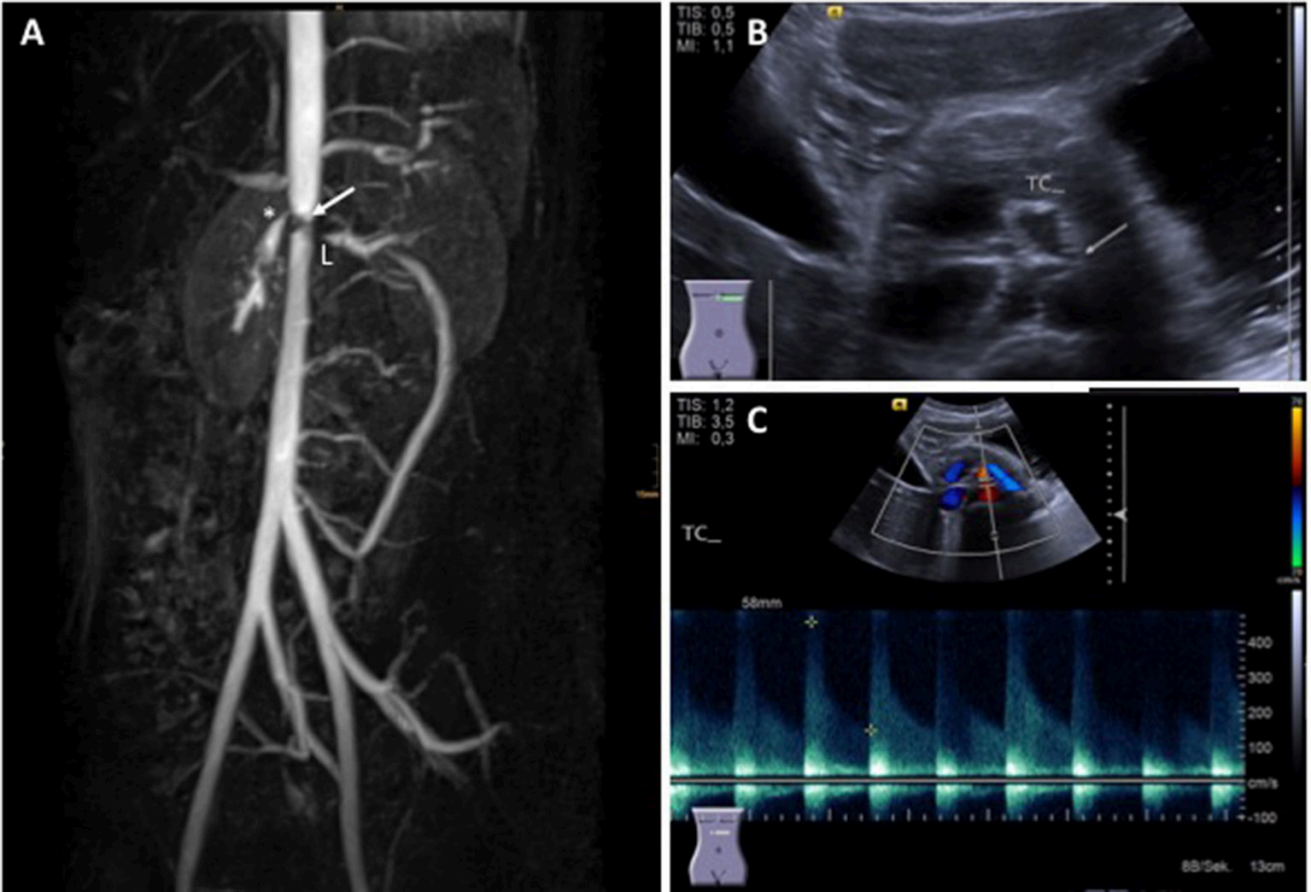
Takayasu arteritis in a 12-year-old girl. (A) MRI angiography showing significant stenosis of the coeliac truncus (*) and lower aorta (→); artifacts are caused by stent implants in renal arteries (L) (provided by Dr. Gabriele Hahn, Pädiatrische Radiologie, Universitätsklinikum Carl Gustav Carus, TU Dresden), (B) transversal abdominal ultrasonography indicating significant stenosis of the coeliac truncus, (C) Stenosis of coeliac truncus resulting in pathologically increased arterial blood flow velocity (provided by Dr. Heike Taut, Klinik und Poliklinik für Kinder- und Jugendmedizin, Universitätsklinikum Carl Gustav Carus, TU Dresden). Consent of the patient was obtained to publish these images.
